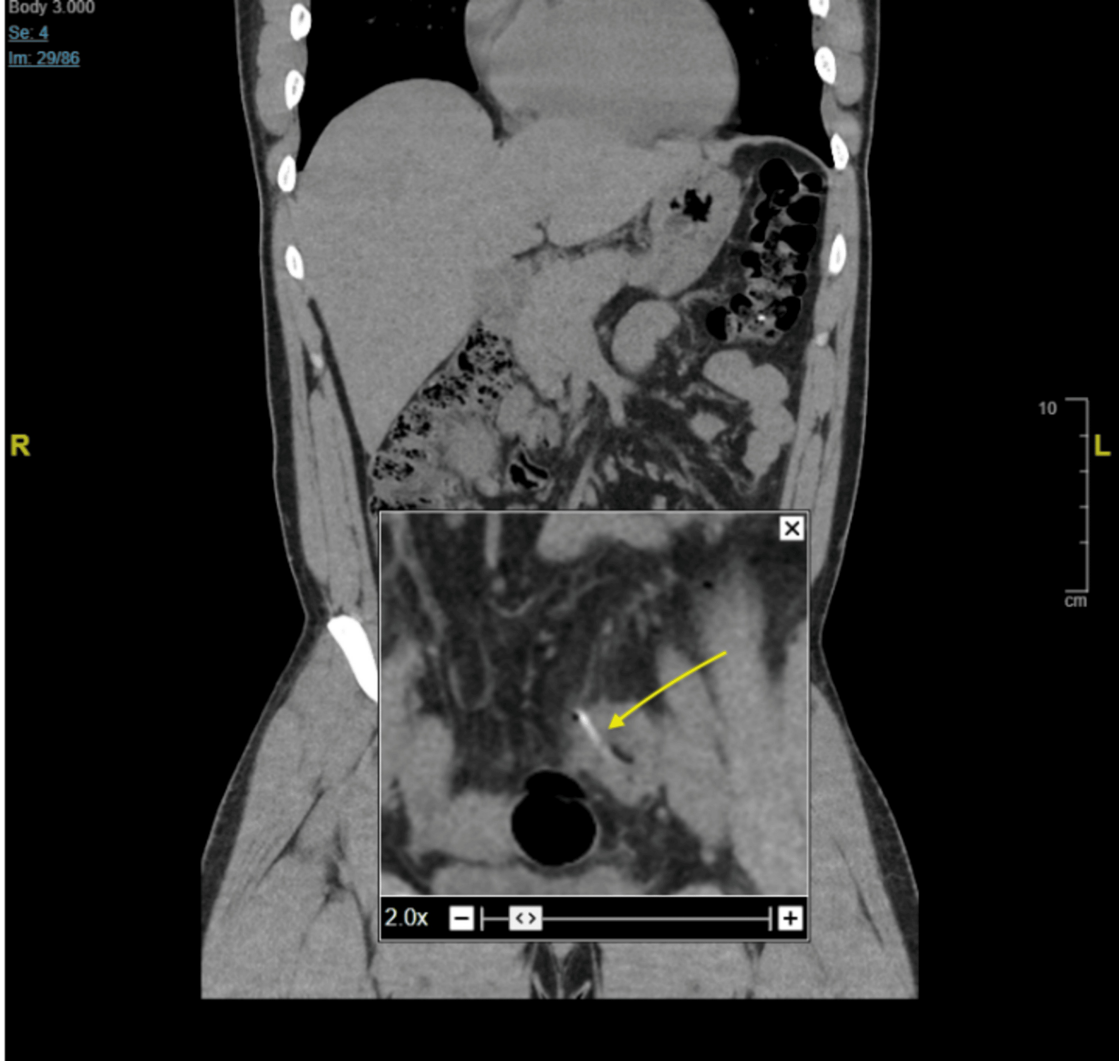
CT abdomen/pelvis without contrast showing a radio-opaque foreign object in the sigmoid colon perforating the bowel wall visualized by the yellow arrow.
